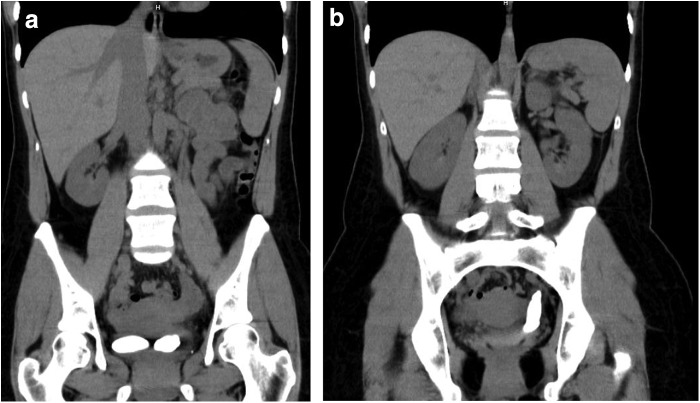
CT scan during work-up revealing (a) large bladder stone as well as (b) 6 cm stone extending up distal left ureter with duplicated left collecting system.
